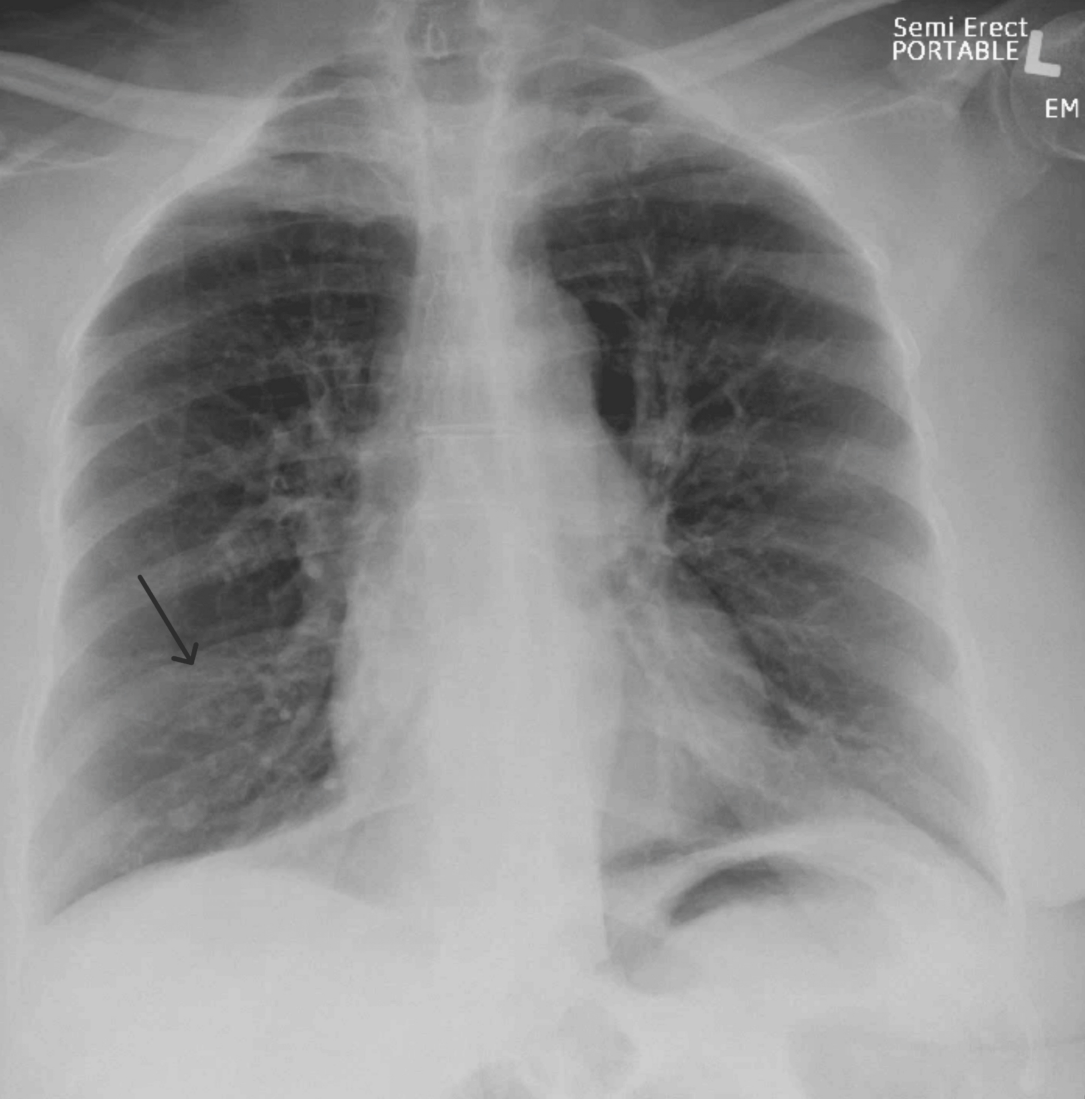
AP chest X-ray Mild bilateral peribronchial cuffing and prominent left lower lobe peribronchial markings were observed. These findings were suggestive of a small airway process without a dense focal infiltrate to suggest bacterial pneumonia (marked by a black arrow). AP: Anterior-posterior.
